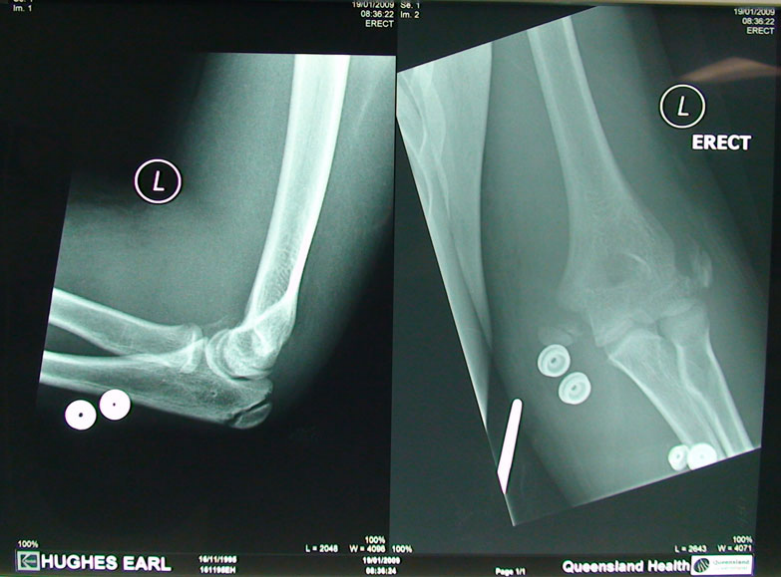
Post reduction X-ray (showing reduced elbow with displaced biepicondylar fractures).
